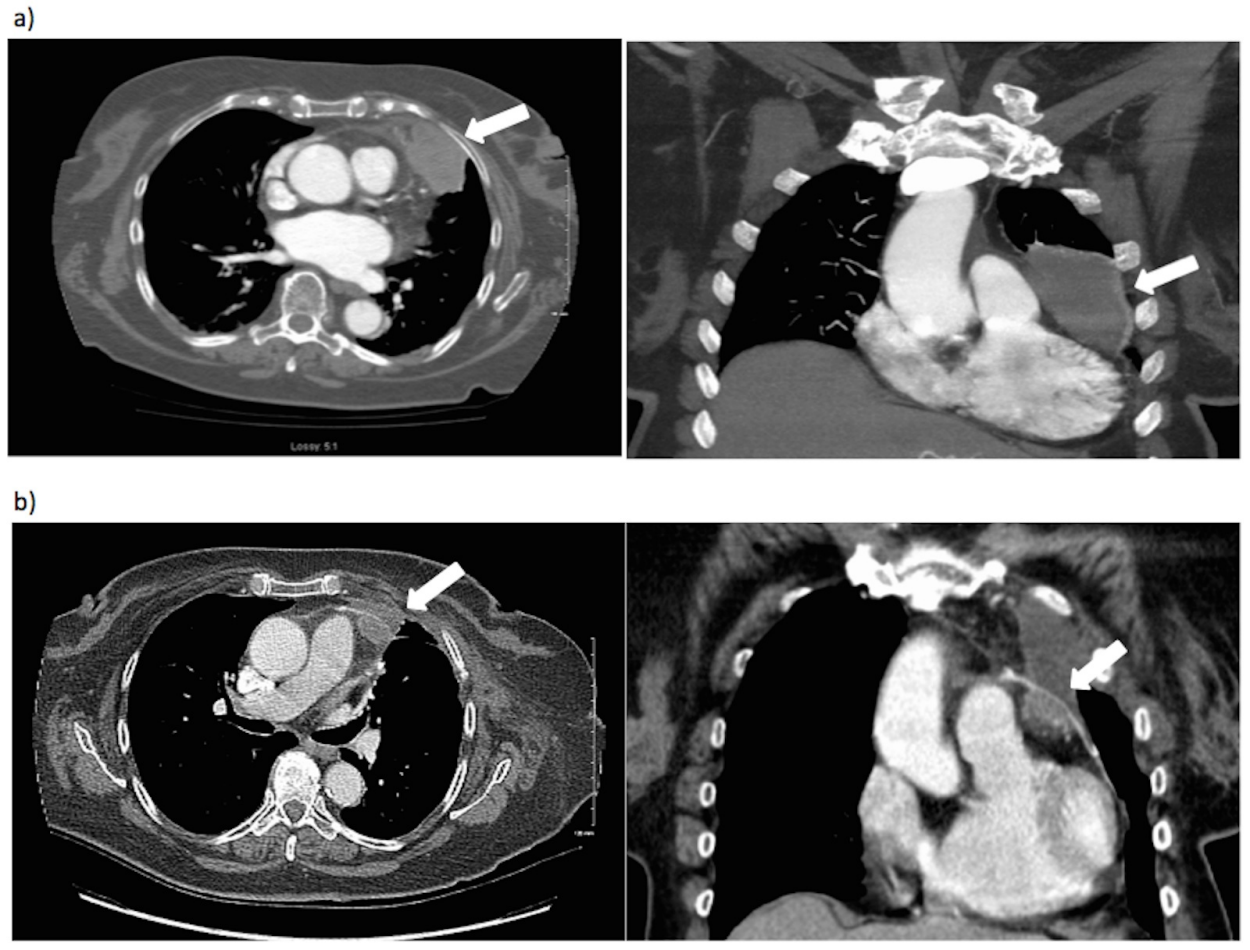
Axial and coronal computed tomography scans of the lesion (a) Pre-surgical resection, July 2011; (b) Post-surgical resection, September 2011.
